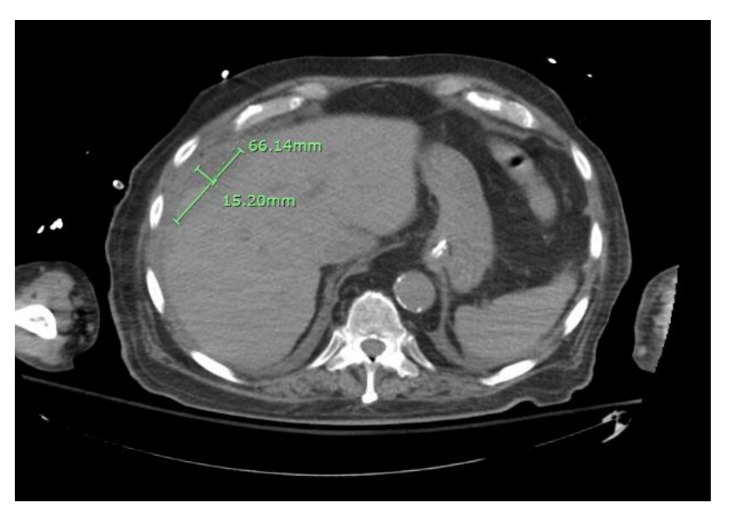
CT abdomen showing reduced size of the right sub-diaphragmatic perihepatic collection.
